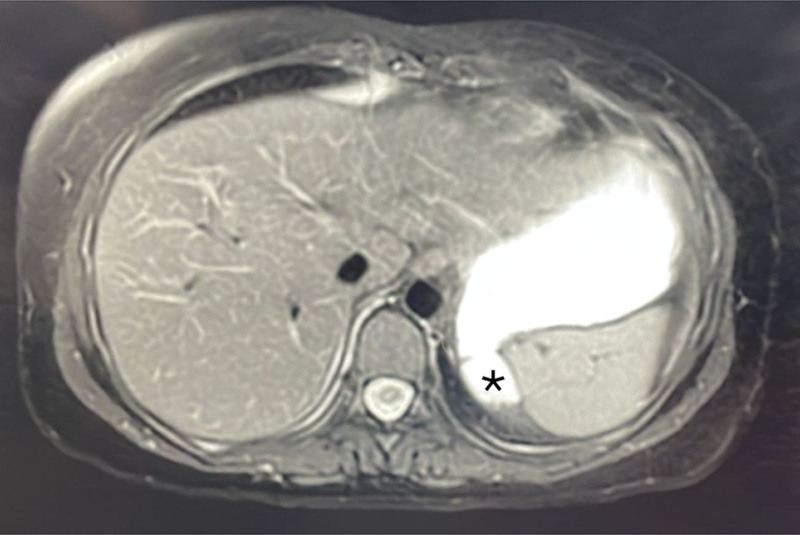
Abdominal MRI: The asterisk designates the gastric diverticulum at the level of the posterior fundus. MRI = magnetic resonance imaging.
